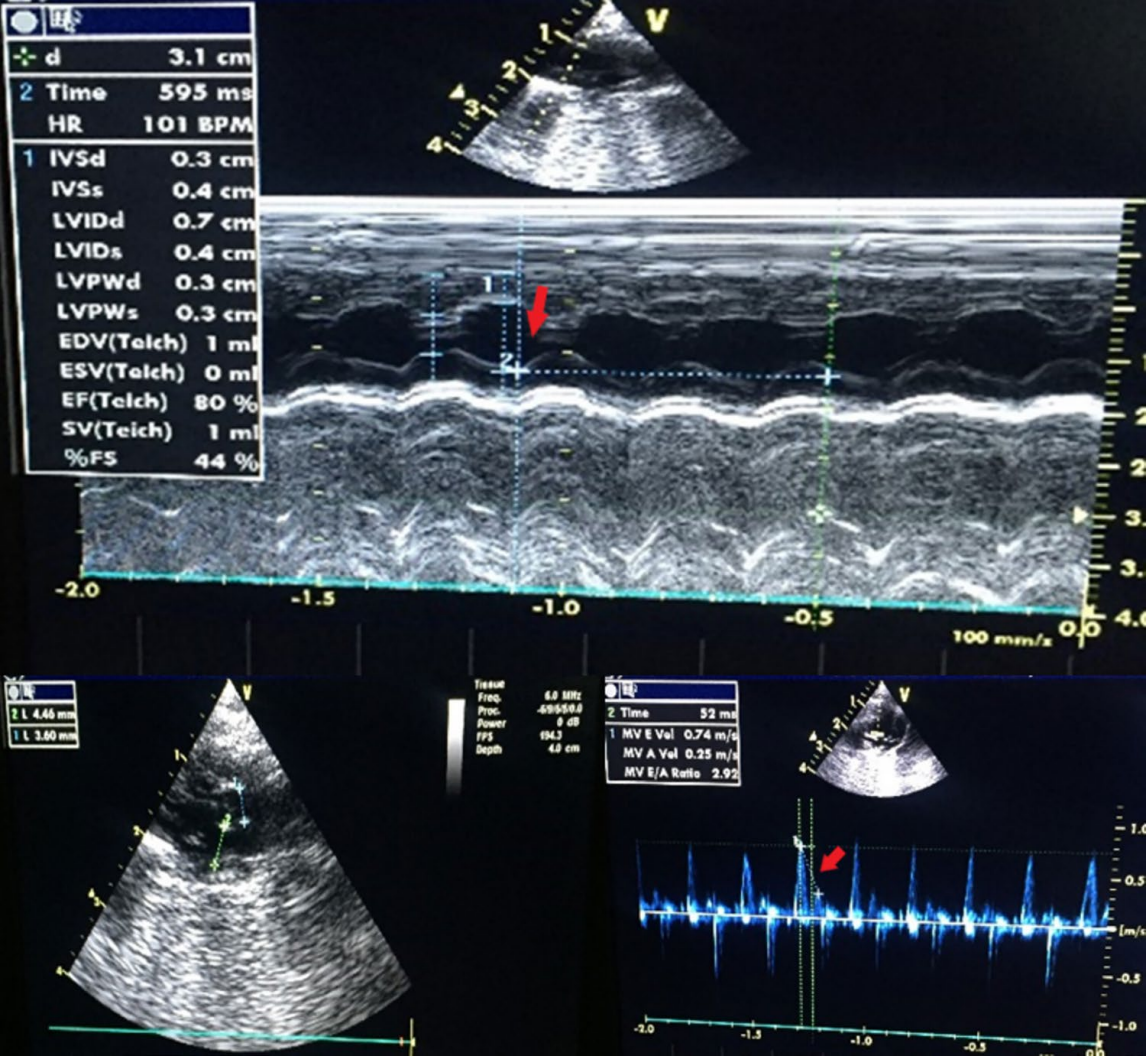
M-mode (top) and two-dimensional (left bottom) echocardiographic images and Doppler tissue images (right bottom) of the left ventricle in Control group. *IVSd* interventricular septal end diastole, *IVSs* interventricular septal end systole, *LVIDd* left ventricular internal diameter end diastole, *LVIDs* left ventricular internal diameter end systole, *LVPWd* left ventricular posterior wall thickness in diastole, *LVPWs* left ventricular posterior wall thickness in systole, *EDV* end-diastolic volume, *ESV* end-systolic volume, *EF* ejection fraction, *SV* stroke volume, *FS* fractional shortening, *MV E Vol* mitral valve flow E-wave, *MV A Vol* mitral valve flow A-wave
